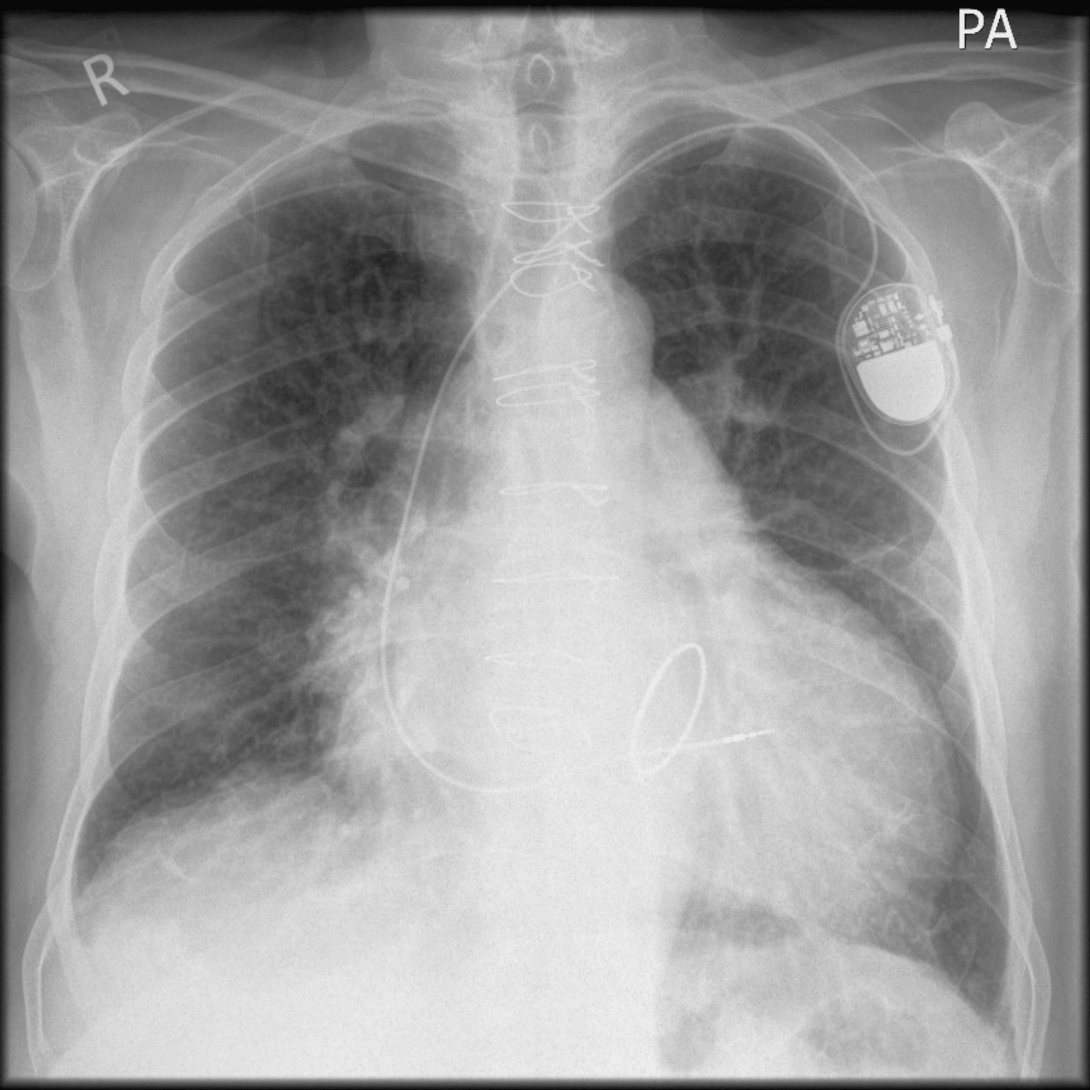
Chest X-ray displaying cardiomegaly and pulmonary congestion It also shows the mitral annuloplasty ring and sternotomy wires from previous surgical repair, along with a single-lead permanent pacemaker
